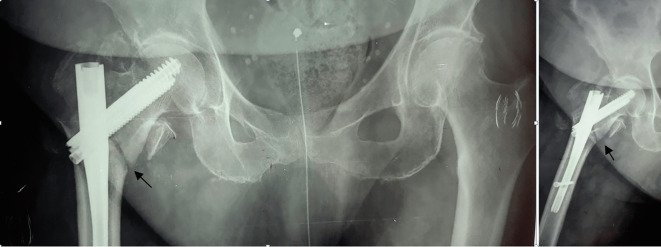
Final X-rays of unstable peritrochanteric fracture treated with cephalomedullary nailing (Trigen InterTAN, Smith & Nephew) and intraoperative compression at the maximum amount permitted.
